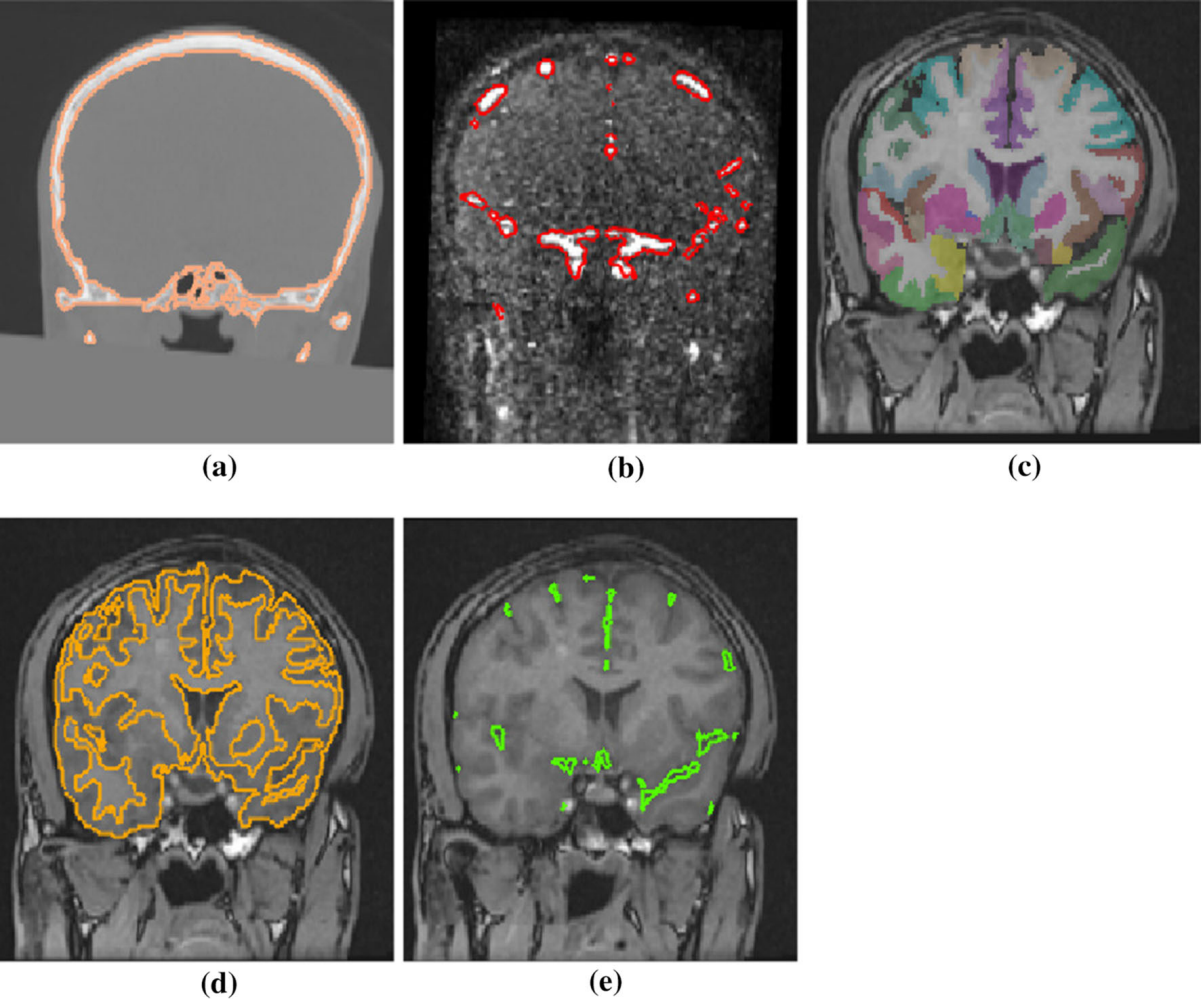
An example patient imaging dataset containing a CT with skull segmentation (*orange*), b MRV with vessel segmentation (*red*), c 3D T1-weighted MPRAGE with brain parcellation, d 3D T1-weighted MPRAGE with GM segmentation (*orange*), and e 3D T1-weighted MPRAGE with sulci segmentation (*green*)
